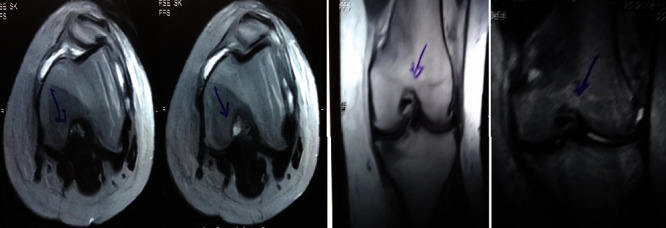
Pretreatment MRI showing grade III tear in the posterior horn of the medial and lateral menisci.
